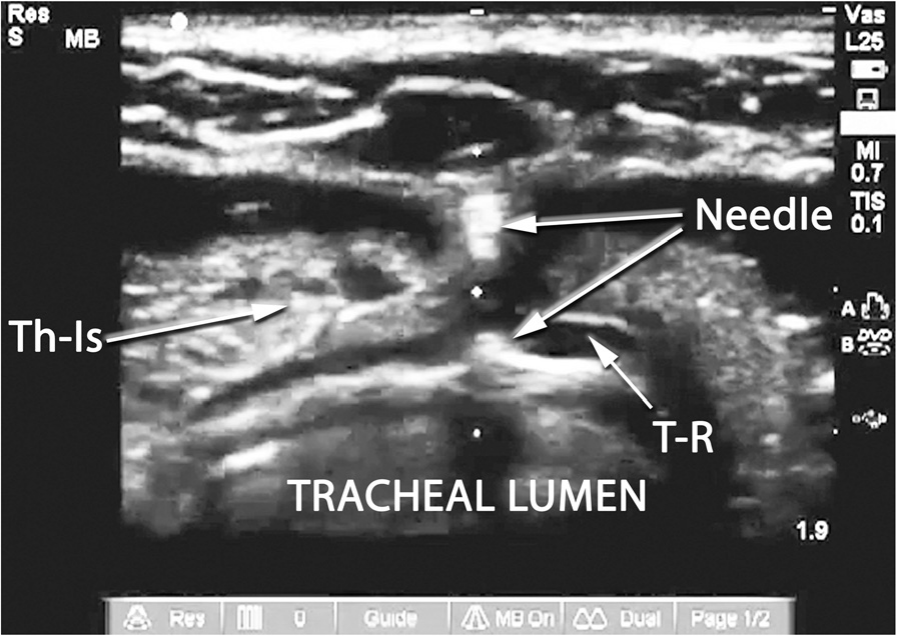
Anterior tracheal wall puncture on ultrasound. Axial view of the trachea demonstrating the visualized part of the needle at the anterior tracheal wall; Th-Is, thyroid Isthmus; T-R, tracheal Ring.
